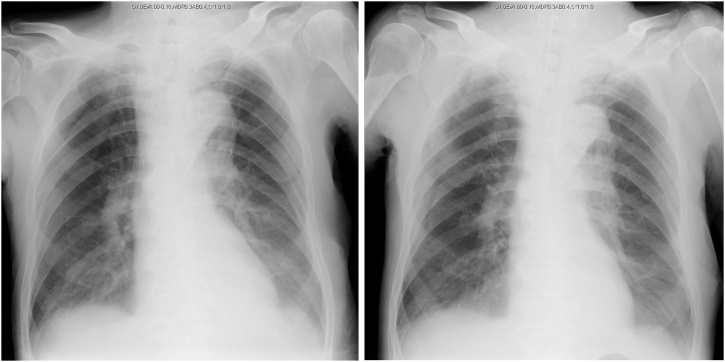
X-rays one day before pulmonary failure (left) and right after the episode (right).
